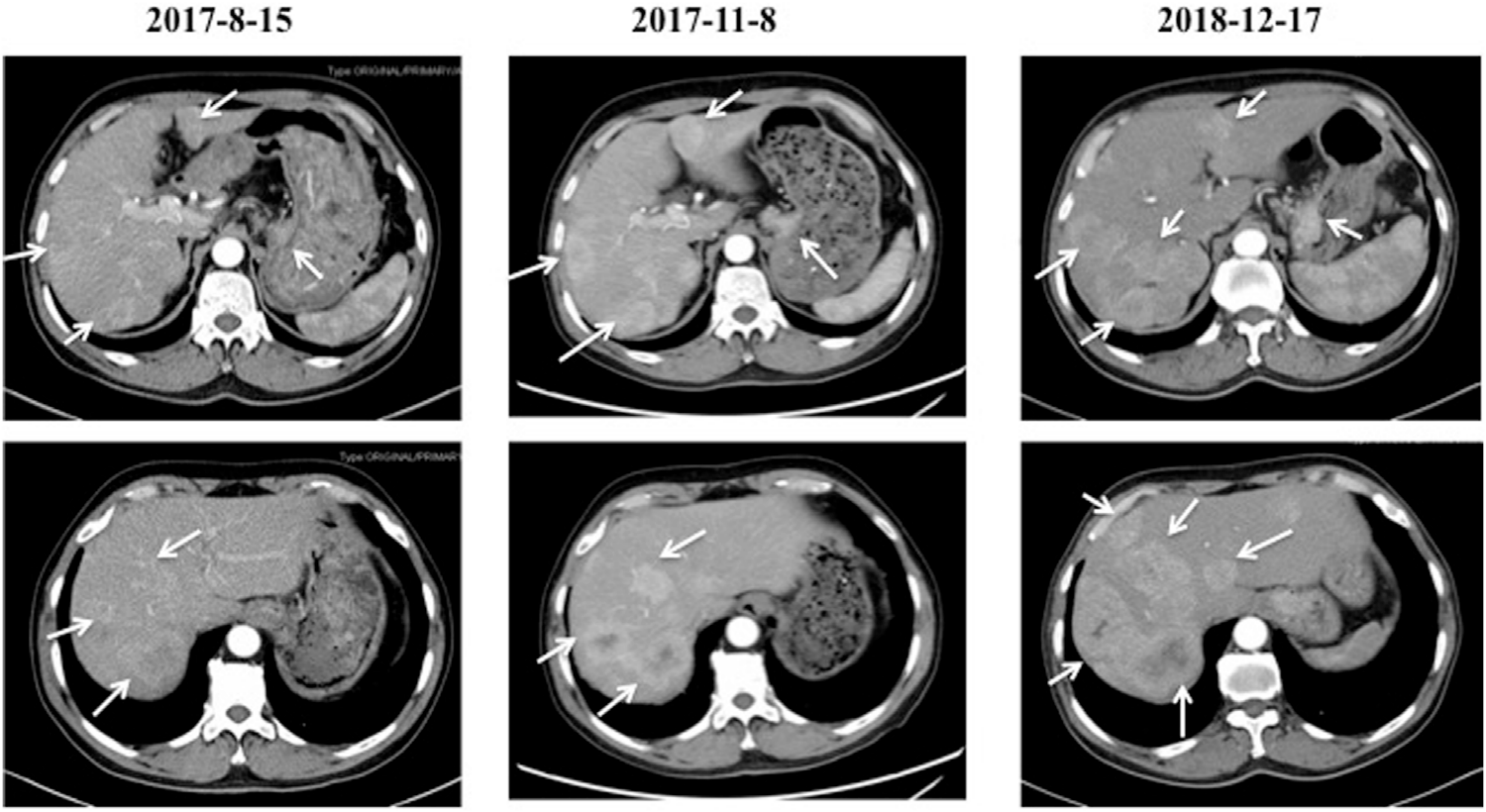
The CT images of the cardiac cancer and hepatic metastases before and after first-line chemotherapy. The cardiac tumor with multiple hepatic metastases were indicated on 15 August 2017. After two cycles of first-line chemotherapy with oxaliplatin and S-1, the cardiac and hepatic lesions showed stable disease (SD) on 8 November 2017. Five months after first-line treatment, the cardiac and liver masses were progressed on 17 December 2018.
